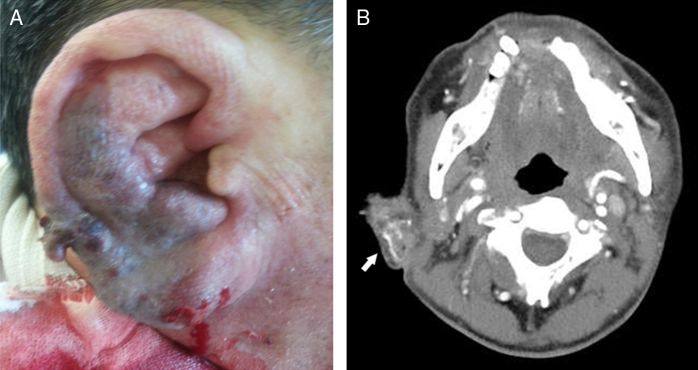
Gross findings and temporal bone computed tomographic angiography findings on the ear of a 60 year-old man, as recorded in the emergency room: (A) the patient presented with a swollen ear and spontaneous massive bleeding; (B) temporal bone computed tomographic angiography revealed a right-side, auricular vascular tangled lesion (white arrow).
